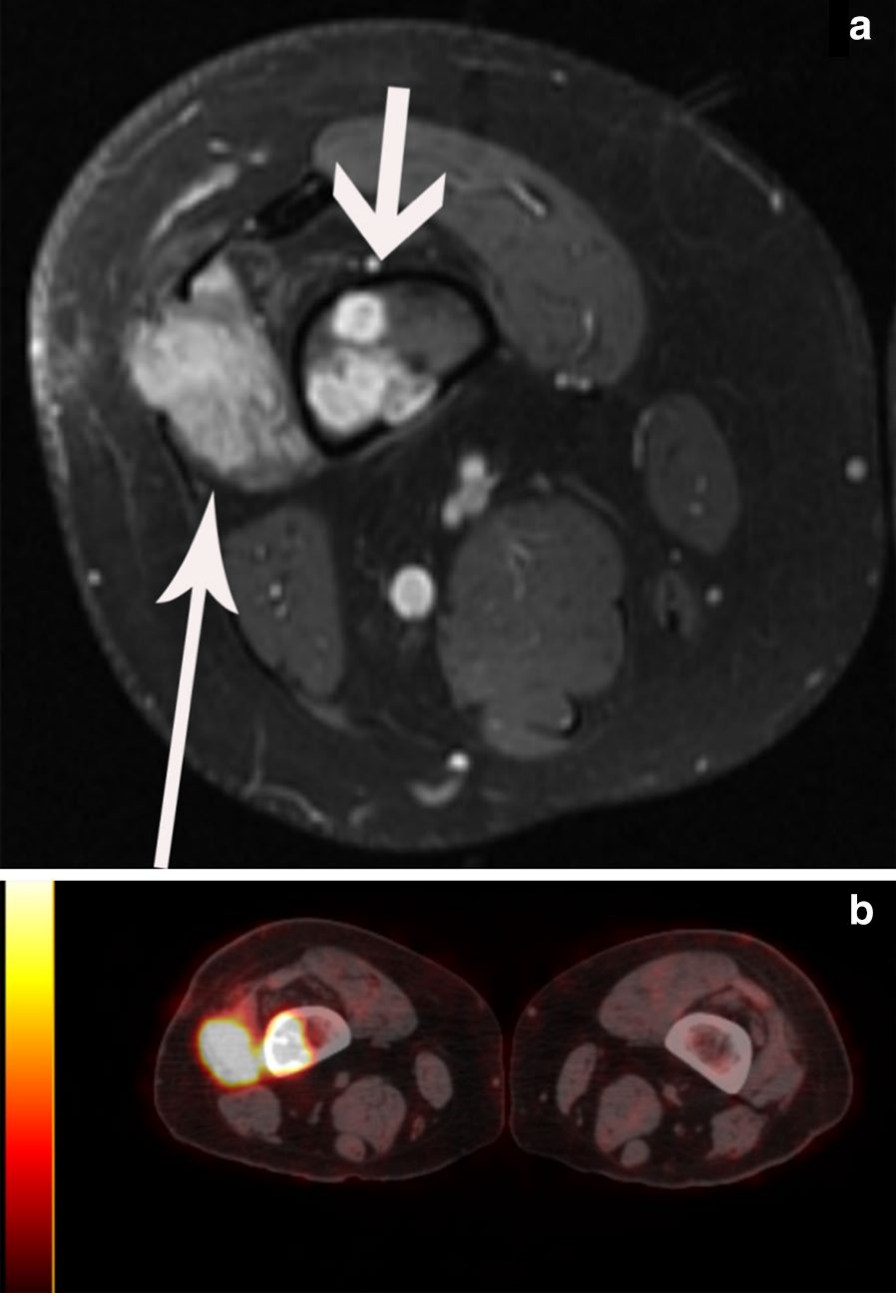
Baseline magnetic resonance imaging (MRI) and positron emission tomography-computed tomography (PET-CT) studies for a 22-year-old man (“Case 2”) with pseudomyogenic hemangioendothelioma. a Baseline contrast-enhanced, fat-saturated T1-weighted axial MR image of the right thigh demonstrates multifocal enhancing lesions in the distal femoral metadiaphysis (*short white arrow*) and vastus intermedius muscle and subcutaneous fat. b PET-CT fusion image through the distal femurs demonstrates corresponding hypermetabolic activity. Restaging examinations after two cycles of gemcitabine and docetaxel (not shown) did not demonstrate any significant response
